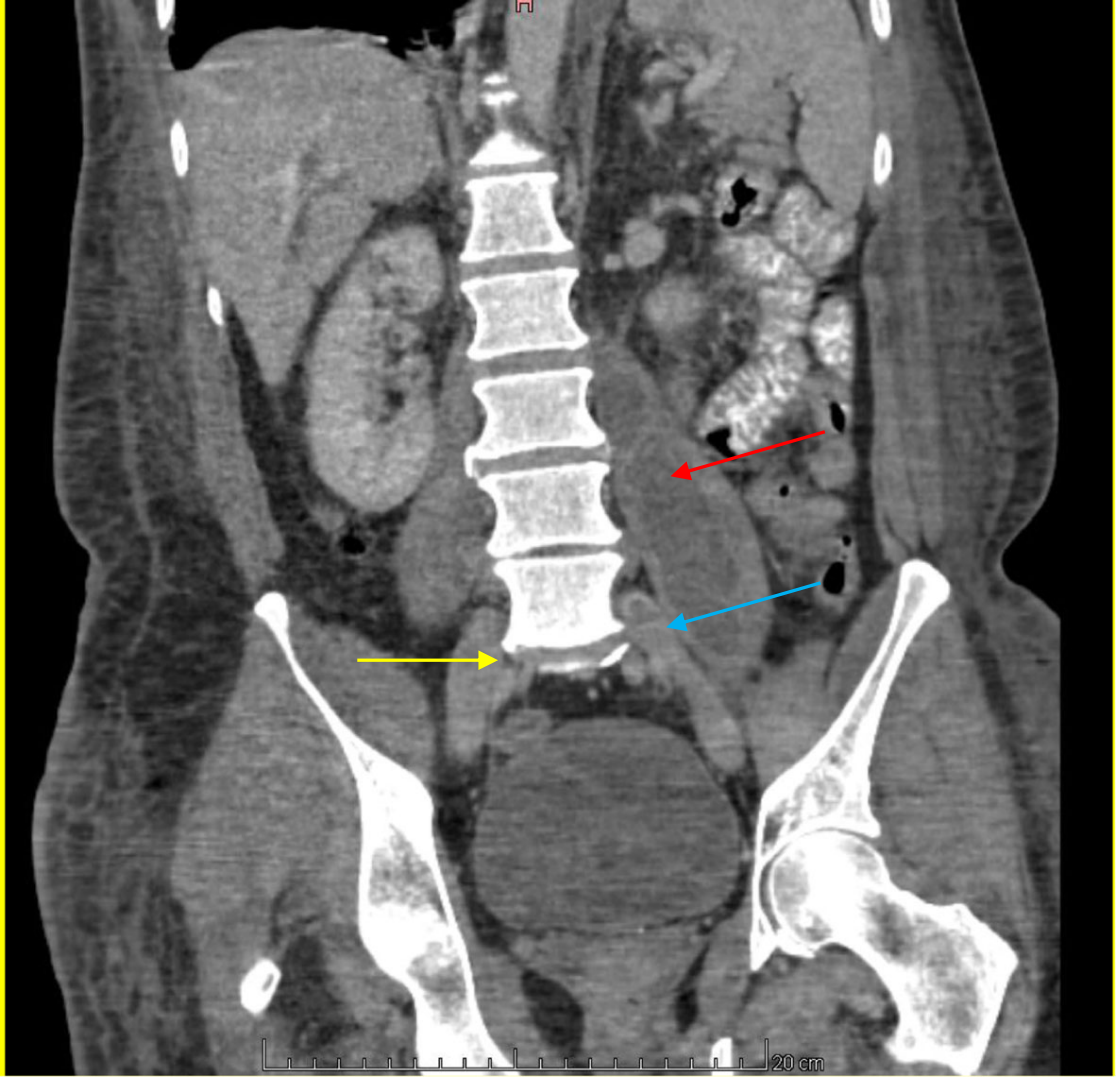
Frontal view of the CT scan. The yellow arrow shows L4-L5 spondylodiscitis, the red arrow shows the left iliac abscess and the blue arrow shows the left iliac thrombosis
